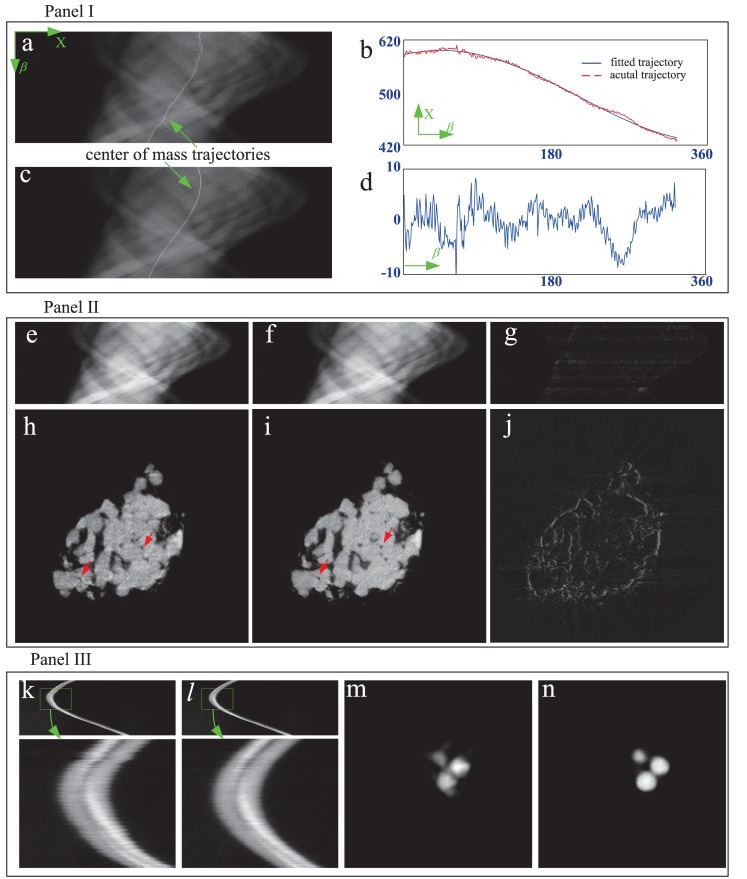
The correction procedure and results for the horizontal shift with the TXM nano-CT experimental data. (a) is the sinogram of the 690th row of the projection with a size 360 × 1024 after the vertical shift correction. (b) depicts the actual trajectory of the center of mass and the fitted one. (c) is the calibrated sinogram and the trajectory of the center of mass using the correction curve in (d). (e) and (h) are the sinogram and the CT image before correction. (f) and (i) are after correction. (g) and (j) show the differences of sinograms and CT images before and after correction. (k) and (m) are the sinogram and the CT image of gold particles before correction. (l) and (n) are after correction.
